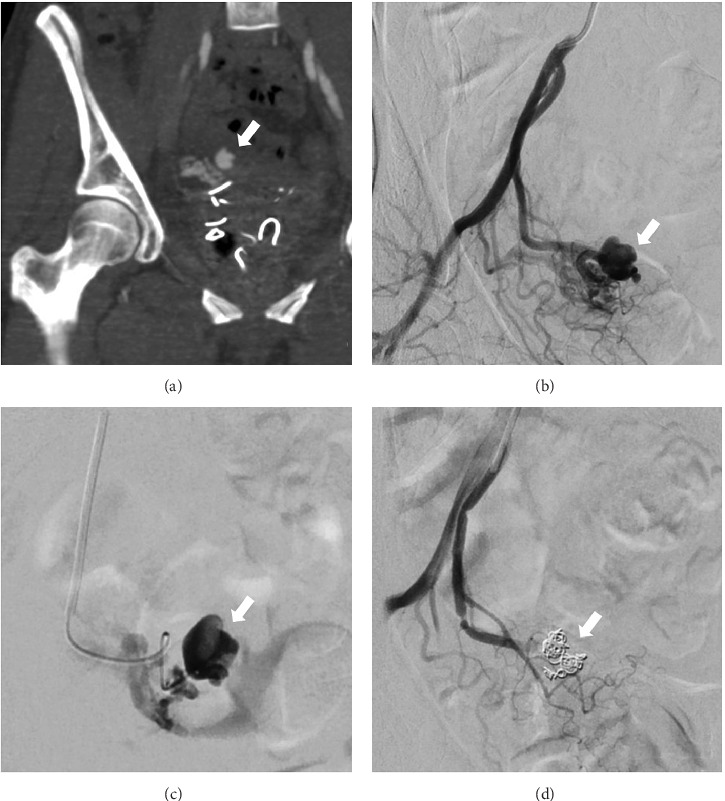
Case 1. (a) CT angiogram showing 10 mm pseudoaneurysm (arrow) arising from the distal right uterine artery. Angiograms show (b) selective catheterisation of the anterior branch of the internal iliac artery and a pseudoaneurysm arising from the right uterine artery (arrow), (c) superselective catheterisation of the right uterine artery and the pseudoaneurysm with associated small arteriovenous fistula (AVF), as seen by the early venous filling, and (d) successful occlusion of the pseudoaneurysm and AVF with coils (arrow).
